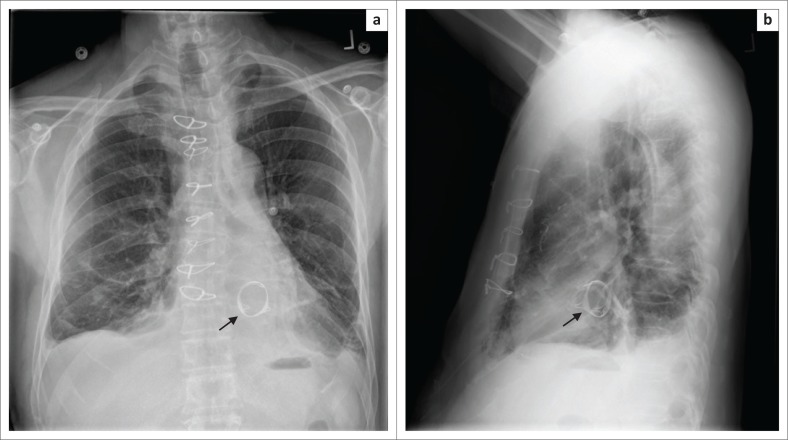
Frontal (a) and lateral (b) chest radiographs showing mitral (perimount tissue) valve replacement (arrow) in a patient with severe mitral stenosis.
